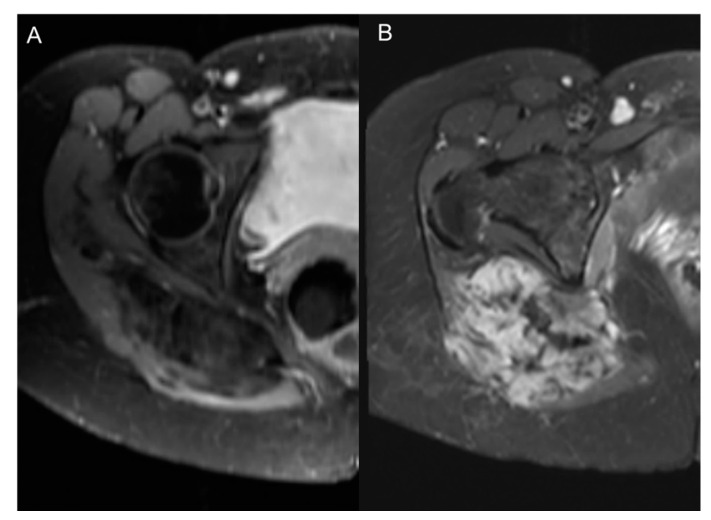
Axial T1 fat suppression contrast-enhanced MRI images of the pelvis in a female patient with fibro-desmoid tumor of the gluteal region. (A) Post- and (B) pre-treatment images after initiation of anti-angiogenic therapy.
